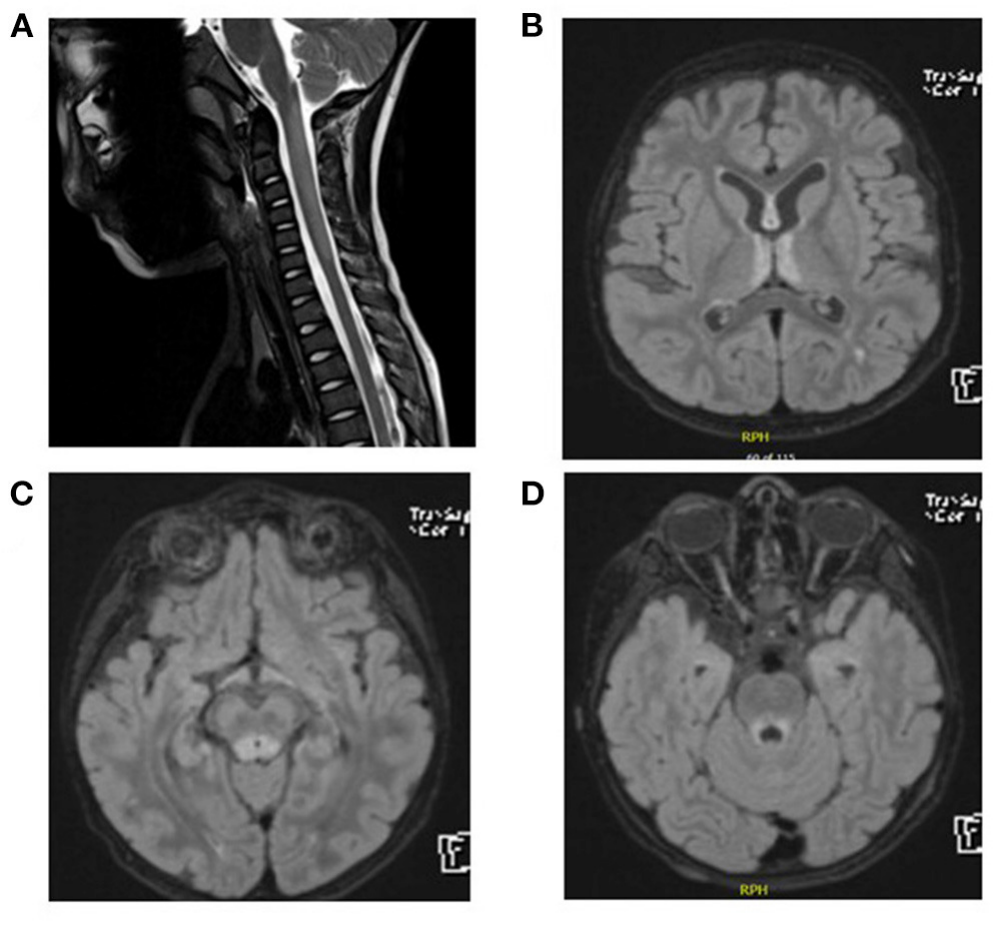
(A) Spinal MRI. Expansile central intramedullary abnormal high T2 signal intensity involving the upper cervical cord extending from the level of the cervicomedullary junction down to the level of the C5 vertebral body. (B–D) Brain MRI and FLAIR images. T2 signal hyperintensities seen on the medial thalami, dorsal aspect of the midbrain, and periaqueductal area of the midbrain.
